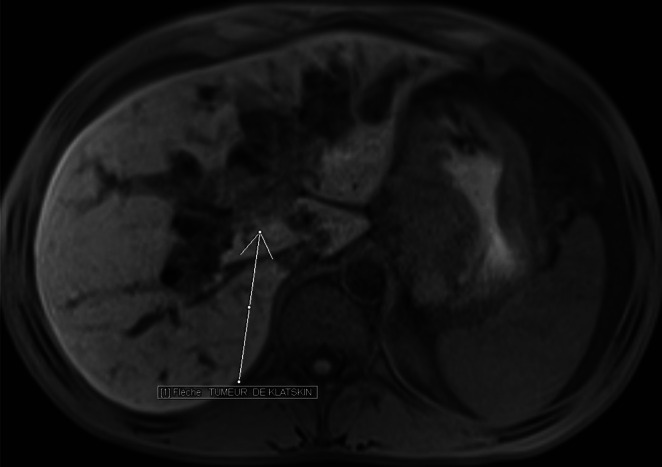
Abdominal MRI T1‐weighted Vibe fs axial section. A voluminous hepatic hilar tissue mass occupying the confluence of the common hepatic duct and the common bile duct is seen in connection with a Bismuth II‐graded extrahepatic cholangiocarcinoma extending to the gallbladder.
